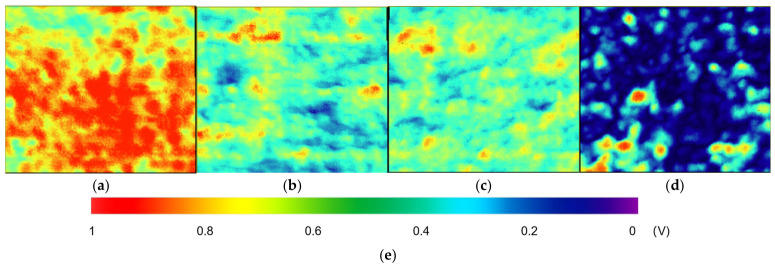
C-scan imaging results of ink wettability of Xuan paper with different thicknesses: (a) single layer; (b) double layer; (c) three layers; (d) four layers. (e) pseudo-color-color bar corresponding to ultrasound amplitude values.
